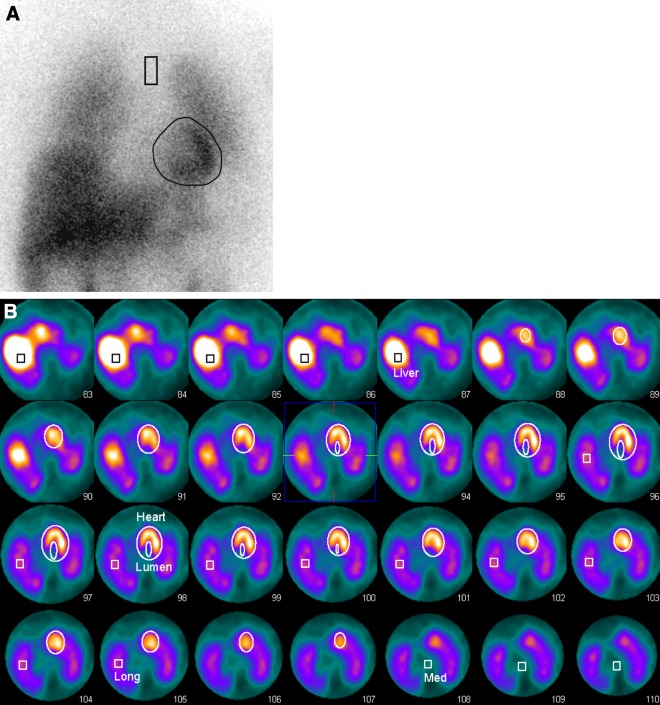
I-123 MIBG acquisition of a patient from the RCT population (age 64 years). This patient has breast cancer for which she receives chemo- and radiation therapy, and has no history of cardiac diseases. A The early planar image shows normal, intense uptake in the cardiac region (eHM = 1.94, dHM = 2.23, WOR = 0.02). B The VOIs for the left ventricle, lumen, mediastinum, right lung and liver are drawn into the SPECT acquisition. Based on these VOIs indices for the HM (eHM = 3.22, dHM = 2.97, WOR = 0.09), MM (eMM = 1.78, dMM = 1.51, WOR = 0.32) and ML (eML = 1.24, dML = 1.04, WOR = 0.82) are determined
